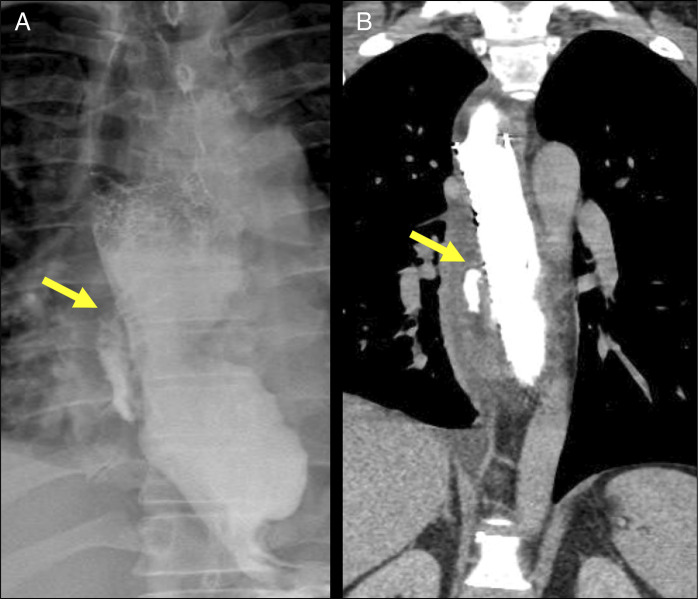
(A) Esophagogram showing a contained leak (arrow). (B) Chest CT with contrast showing a persistent contained leak (arrow).
